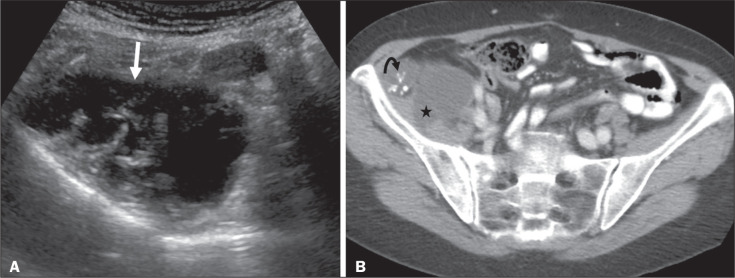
LAMN with an atypical presentation. A: Ultrasound image shows a
